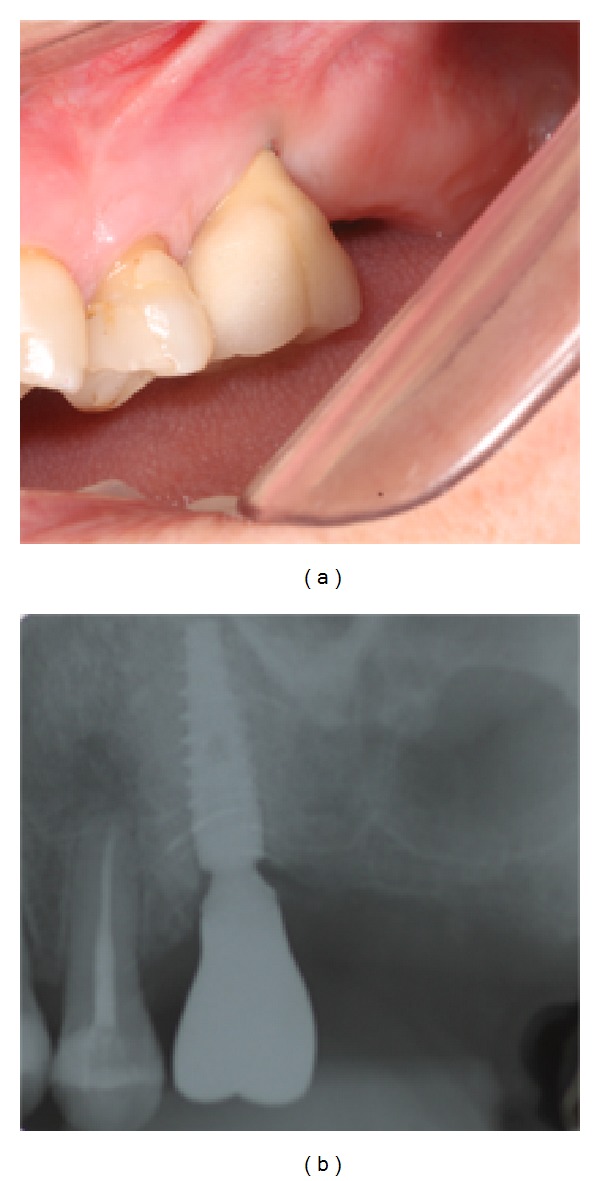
Finalized porcelain-fused-to-metal crown (a) and final X-ray (b).
